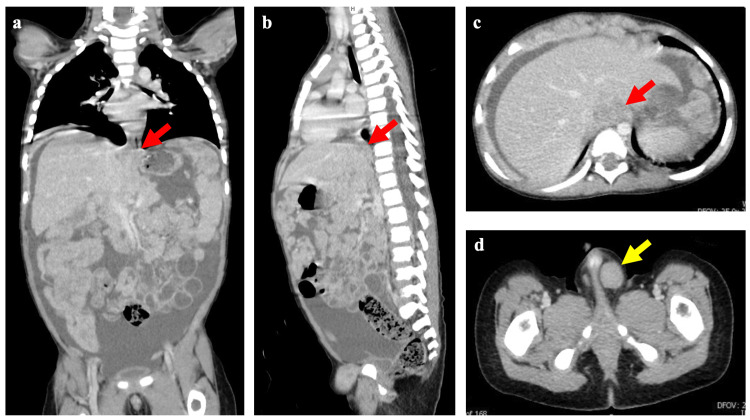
Contrast-enhanced chest and abdominal computed tomographic images. The red arrows indicate a tumorous lesion diagnosed as rhabdomyosarcoma on a coronal image (a), a sagittal image (b), and a horizontal image (c). The yellow arrow indicates a metastatic tumorous lesion of rhabdomyosarcoma on a horizontal image (d). The size of tumors originating from the diaphragm in panel (c) was 20.80 x 19.46 mm, while that of tumors in the left inguinal region in panel (d) was 12.30 x 13.80 mm.
